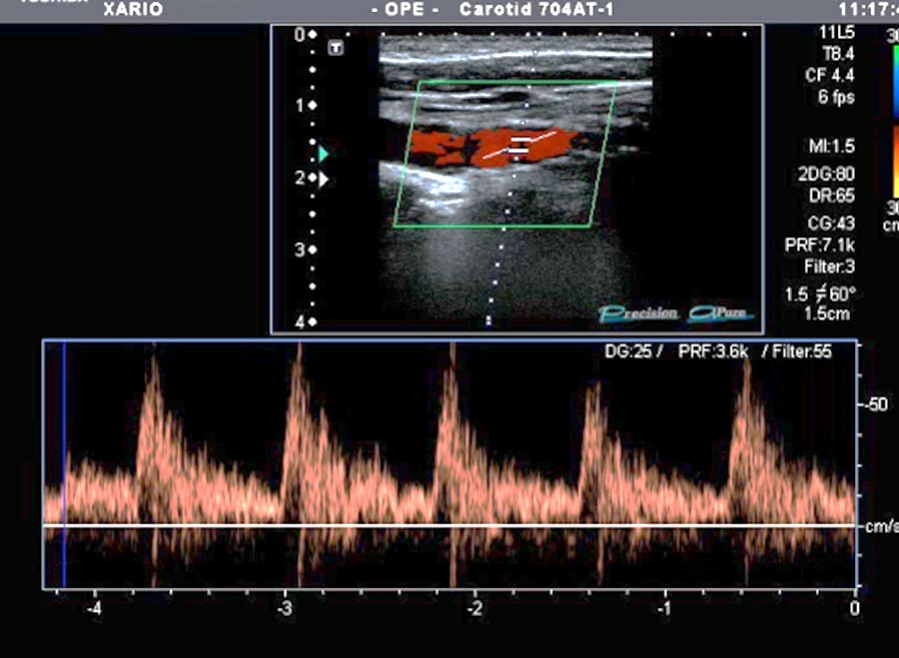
Color and pulsed Doppler demonstrating restored normal flow in common carotid artery at the site of the stent
